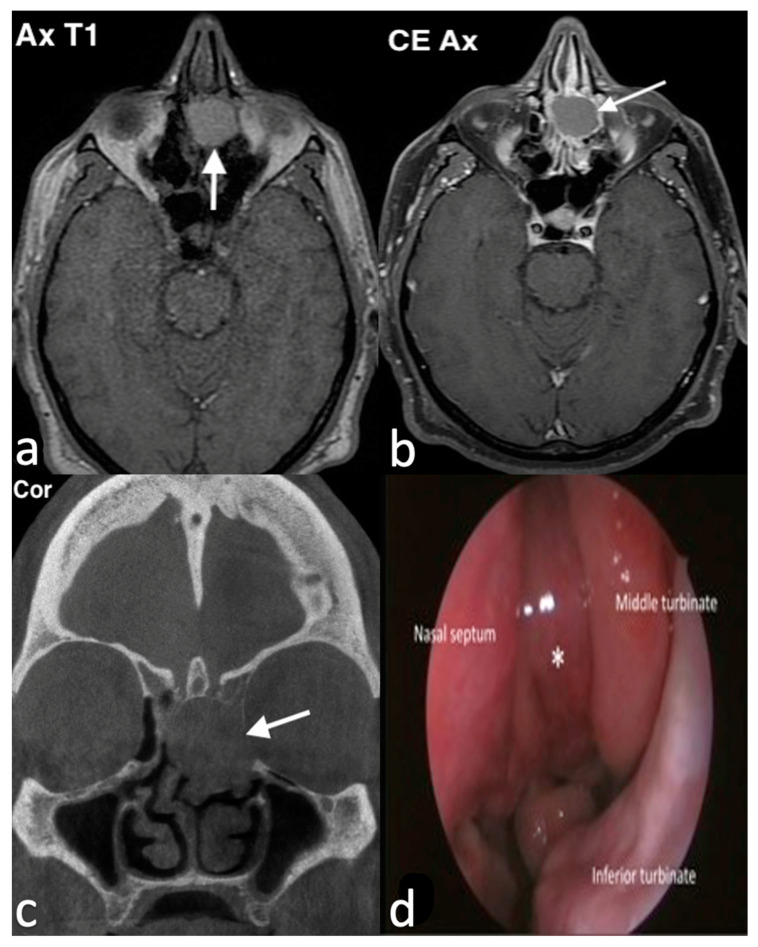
Septal mucocele in a 49-year-old man who had been experiencing long-standing bilateral nasal obstruction. Axial T1 MR image (a) shows an oval mass with well-defined margins, high intensity content; due to fluid-proteinaceous content, in the middle-upper third of nasal cavity the mass causes partial nasal obstruction in both nasal cavities. On axial T1, fat saturation after intravenous Gd (b), the lesion shows peripheral wall enhancement. On coronal CBCT (c), the nasal septum bone is expanded by the mass (arrow), laterally displacing the middle-left turbinate. Endoscopic view (d) of the left nasal cavity shows the mucocele (asterisk) between nasal septum and middle left turbinate.
